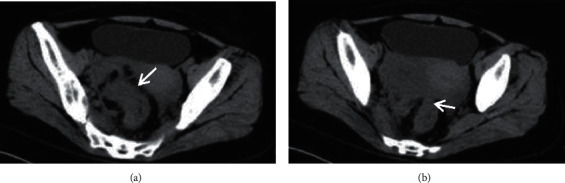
CT images of FPA patients. (a, b) CT plain scan images with a layer thickness of 10 mm and an interval of 2 mm, respectively. There were multiple cord adhesions and irregular mass in the pelvic cavity.
